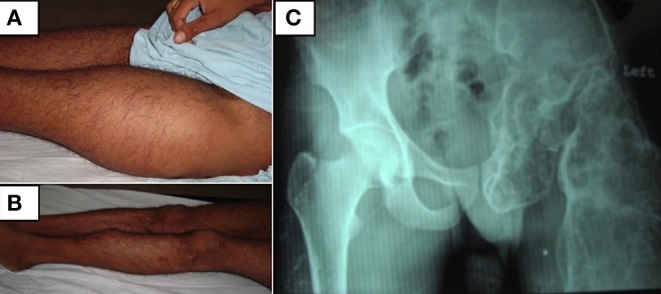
(A,B) Clinical photographs of the patient showing swellings involving the whole of left thigh and anterior part of the left mid-shin. (C) Radiograph of the pelvis showing a grossly deformed left femur and left ischium with multiple expansile lytic areas.
